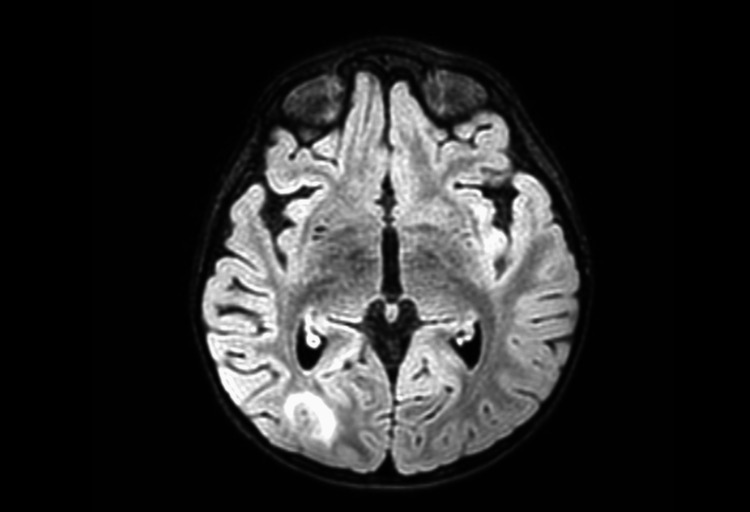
MRI findings consistent with PRES (patient 2) Parieto-occipital high-intensity lesions in the white matter.
